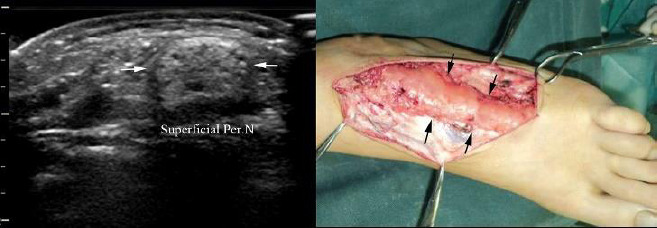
Ultrasonic images and intraoperative conditions of the enlarged superficial peroneal nerve.
